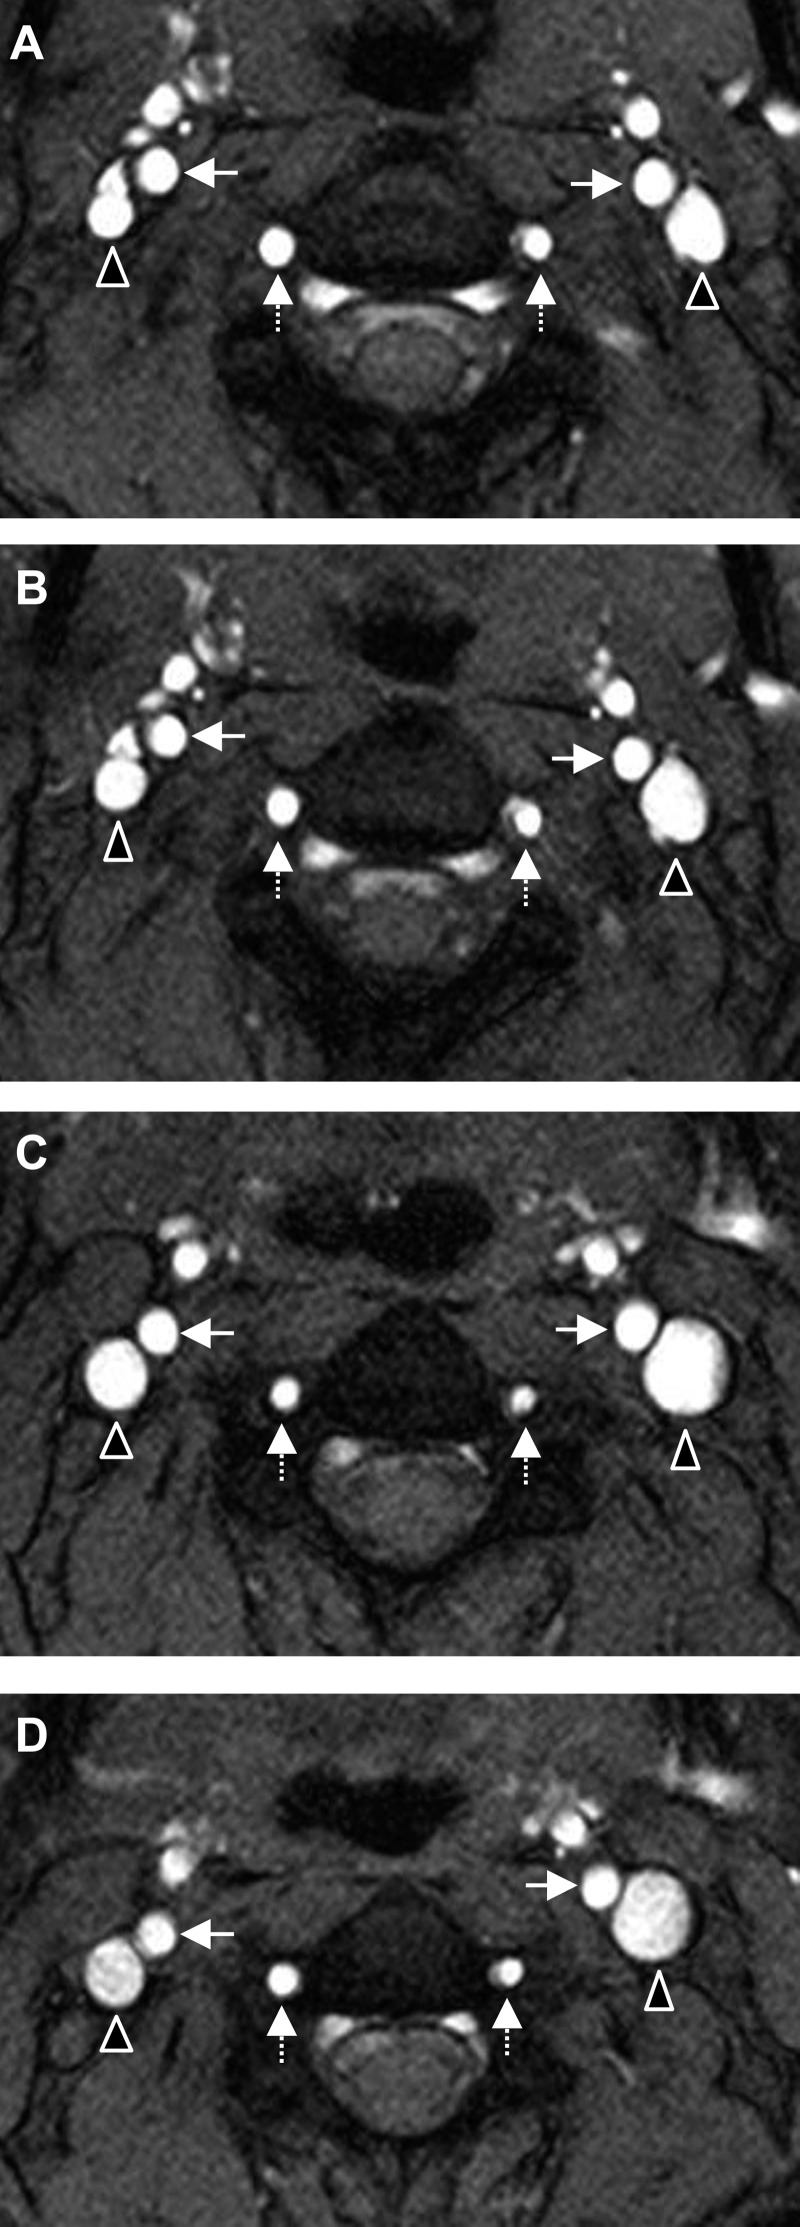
Cross-sectional magnitude images from a phase-contrast MRI sequence taken between the second and third vertebrae in one subject. The images show the left and right internal jugular veins (triangles), internal carotid arteries (solid arrows), and vertebral arteries (dashed arrows) at 0° baseline (*A*), −6° HDT (*B*), −12° HDT (*C*), and −18° HDT (*D*).
